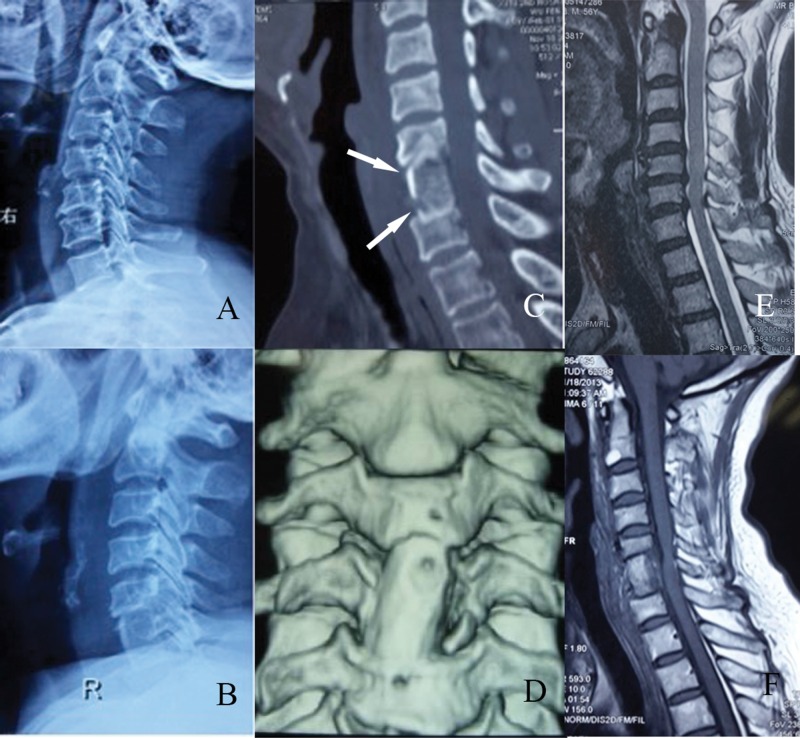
Imaging examinations of a patient who underwent C5-7 fusion. A: Radiograph, lateral view; pre-operation. B: Radiograph, lateral view; post-operation. C: Computed tomography scan, axial view; note the poly L/lactide screws (arrow). D: Three-dimensional reconstruction of the graft. E: Magnetic resonance imaging scan, axial view; pre-operation. F: Magnetic resonance imaging scan, axial view; post-operation.
